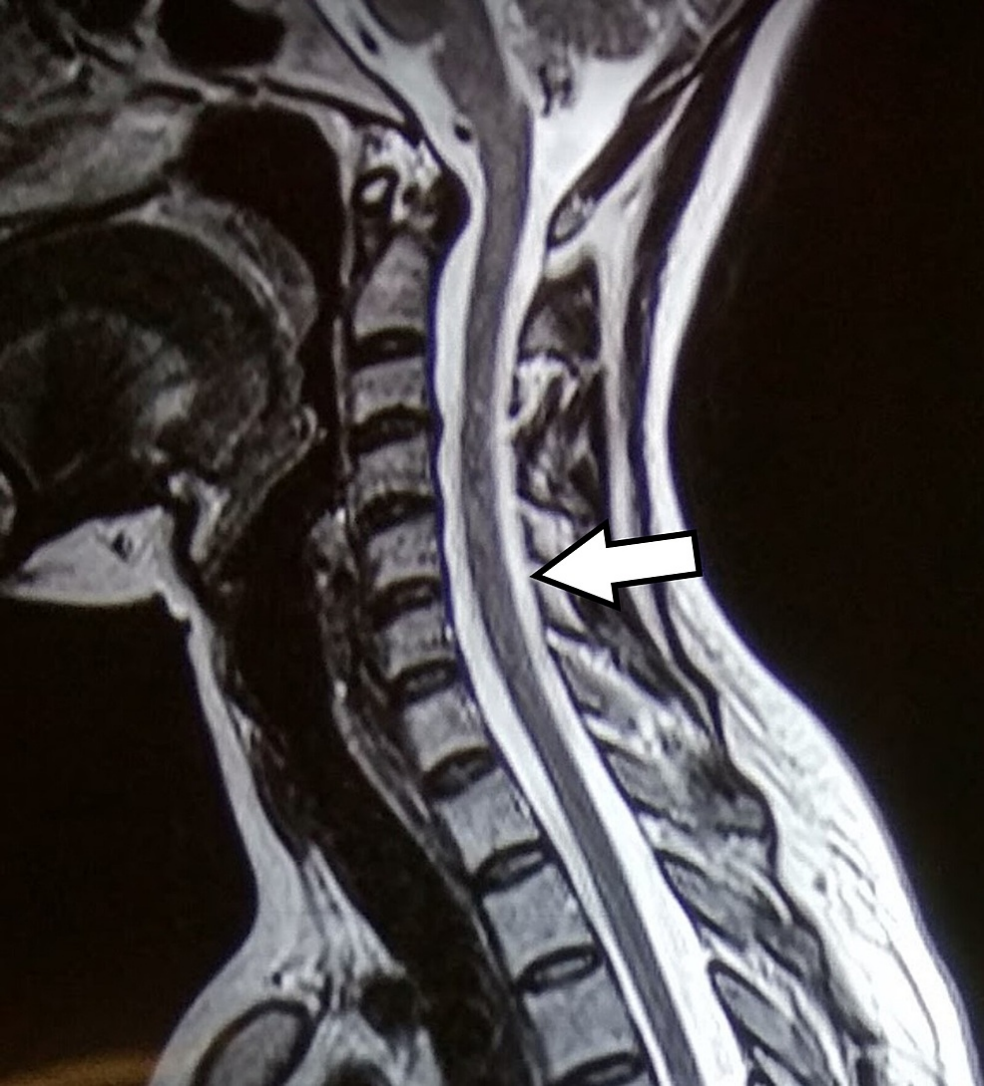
MRI (sagittal view) of the patient showing no obvious compression
